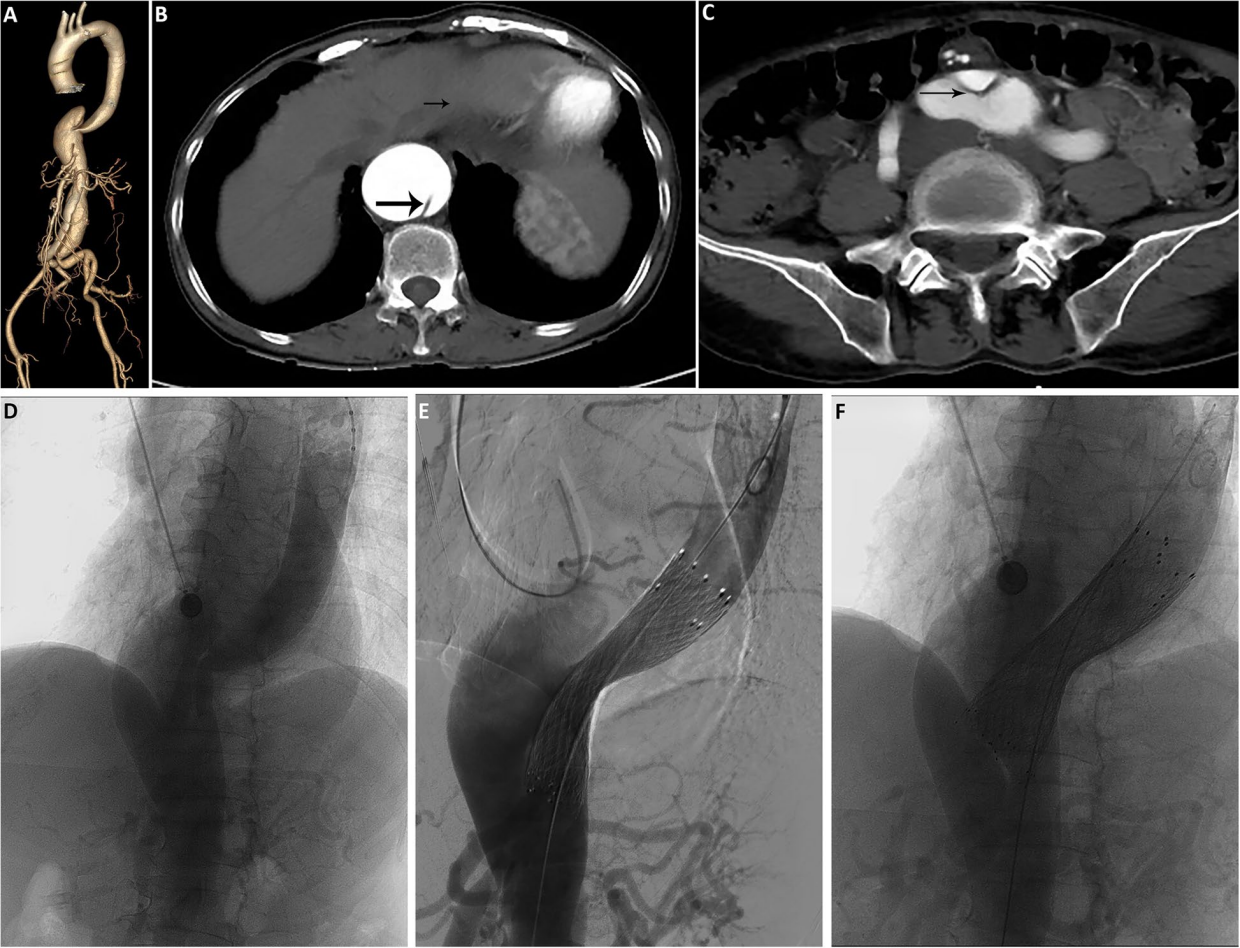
Multi-layer bare metal stents occluded the aortic dissection to reduce blood flow velocity in the false lumen. A-C Preoperative CTA image of the aorta. Arrow shows the intimal flap of aortic dissection. D Preoperative DSA image. E Image of a metal bare stent implanted during the procedure. F Angiogram on completion of the procedure
